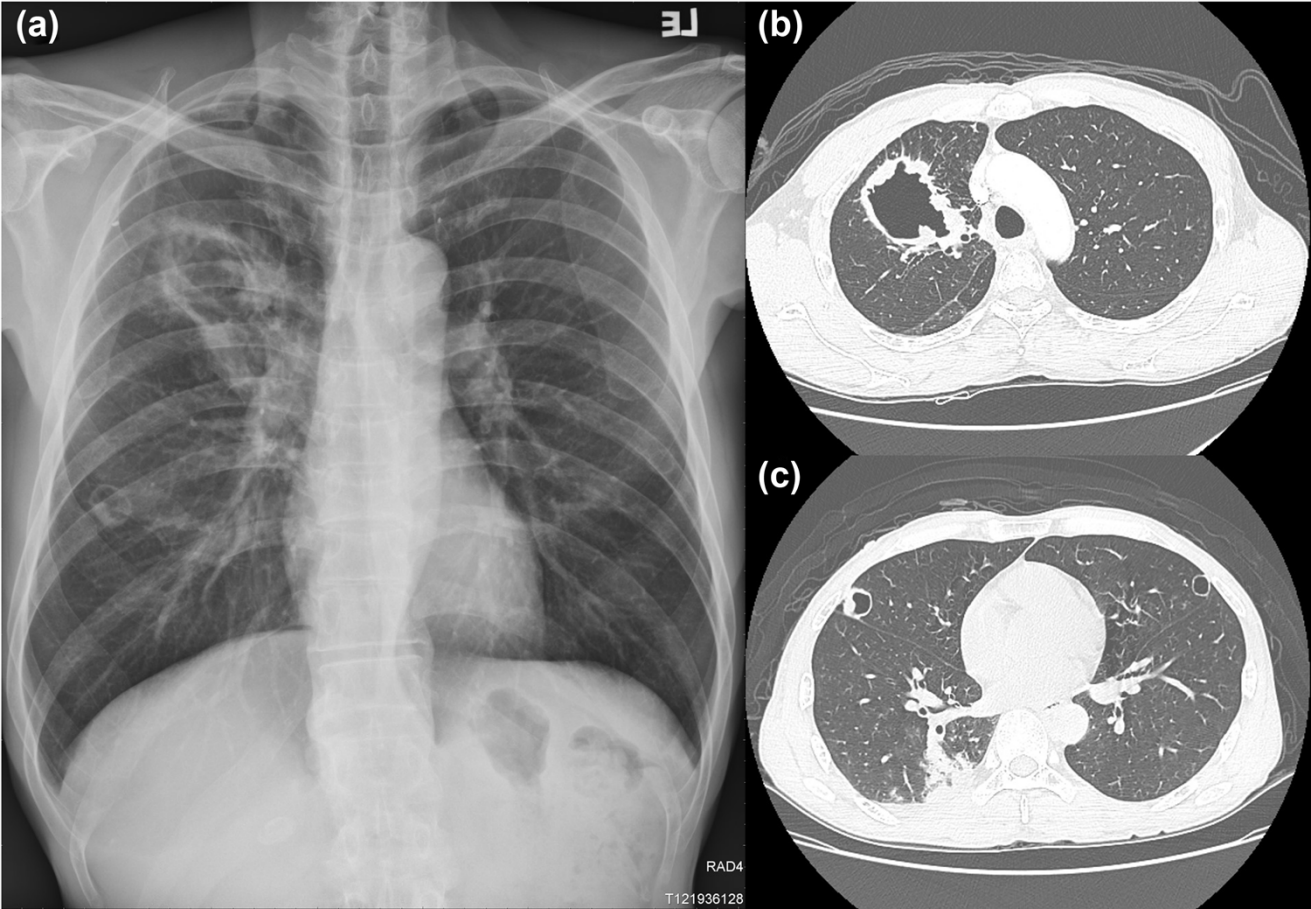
Chest radiography and computed tomography upon initial presentation: (a) RUL cavitary lesion and two cystic lesions within the bilateral upper lung field and (b and c) a cavity with a ball-in-hole lesion in RUL and two small cystic lesions in the bilateral upper lobes.
